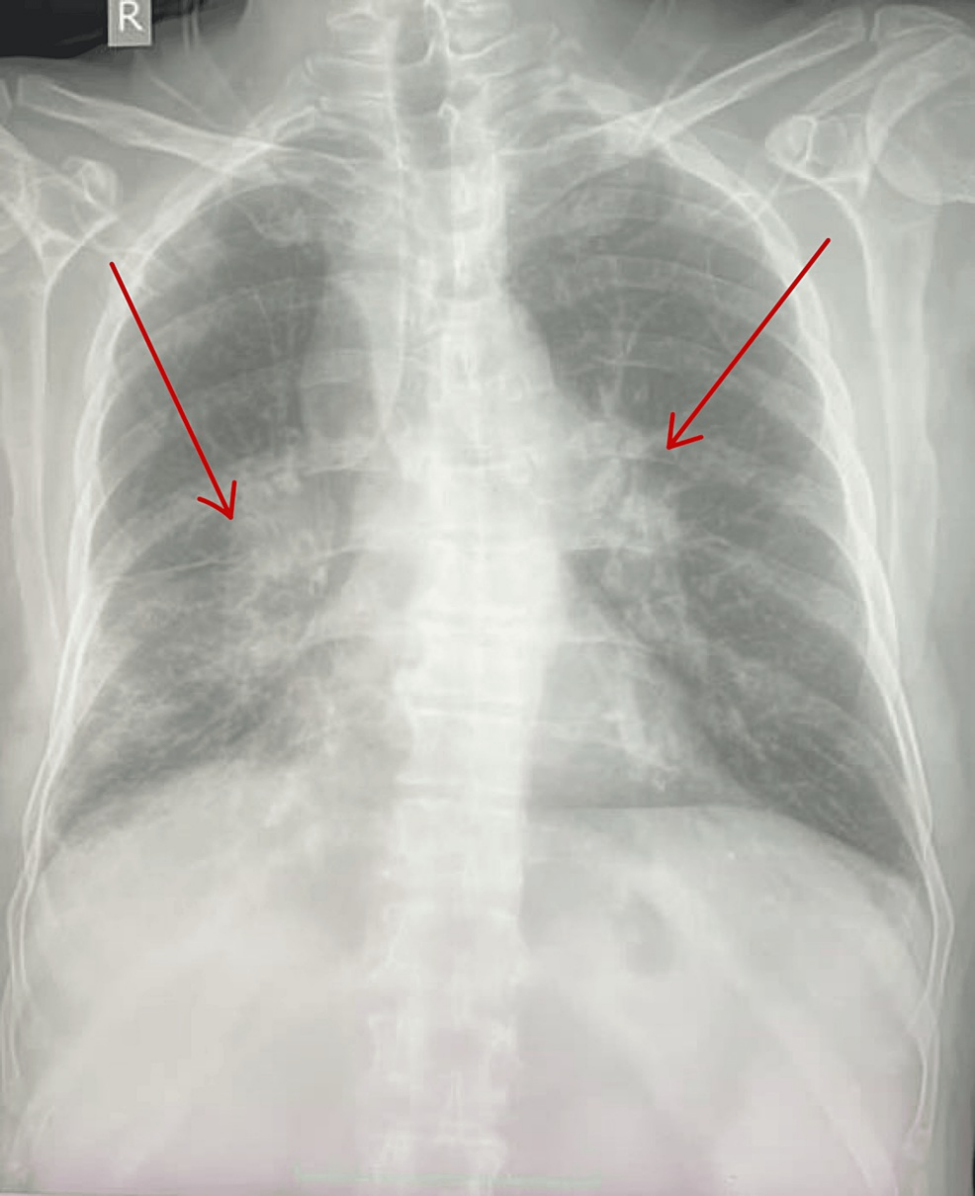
Chest X-ray showing hilar lymphadenopathy *Findings are marked with a red arrow.
